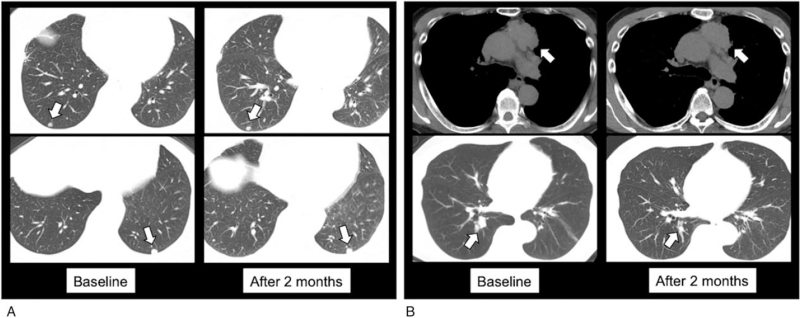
Chest CT scan showing the serial changes at baseline and 2 months after lenvatinib initiation. (A) Chest CT image of Case 1 reveals no remarkable changes in the size of the target lesions (white arrow). (B) Chest CT image of Case 2 presents the decrease in the size of the target lesions (from 80.9–67.1 mm) (white arrow). CT = computed tomography.
